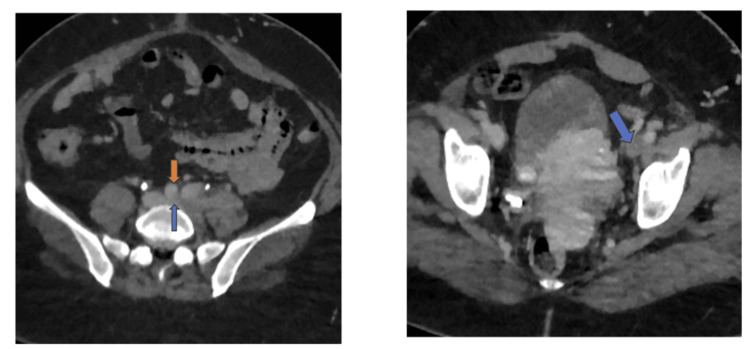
Left: Axial contrast CT showing compression of the left common iliac vein (blue arrow) and right common iliac artery (red arrow). The left common iliac vein appears flattened. Right: Axial CT showing thrombus in the left external iliac vein (blue arrow).
